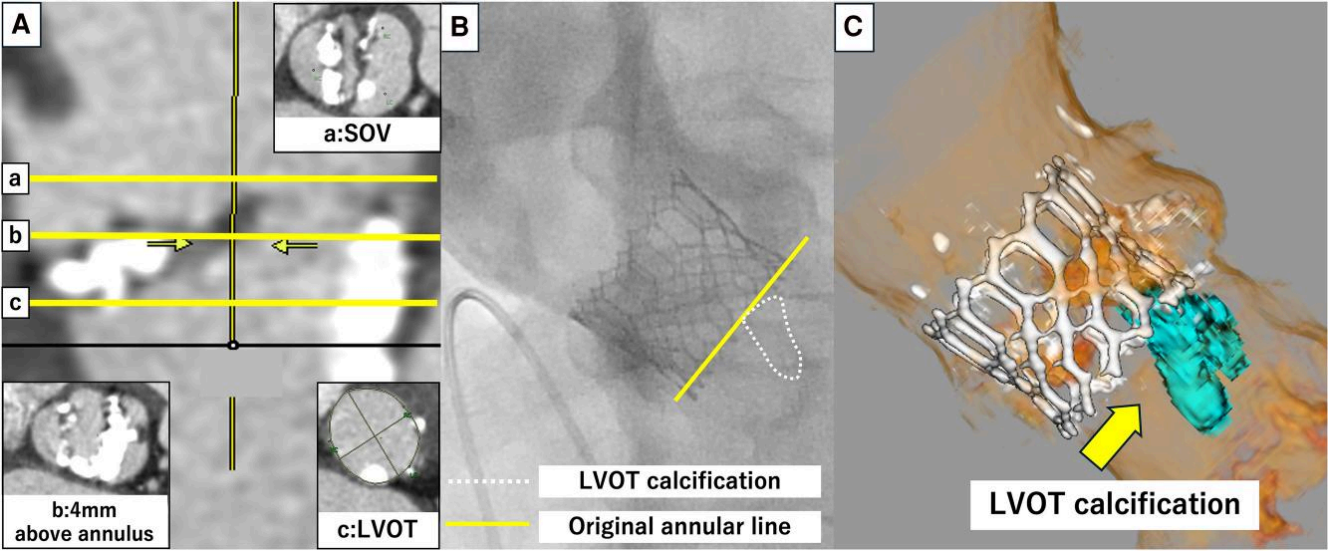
Pre- and post-procedural images of Case 2. (*A*) Pre-procedural computed tomography: a, sinus of Valsalva level; b, 4 mm above the annular level; c, left ventricular outflow tract level. (*B*) Aortography after transcatheter aortic valve replacement. (*C*) Post-procedural computed tomography.
